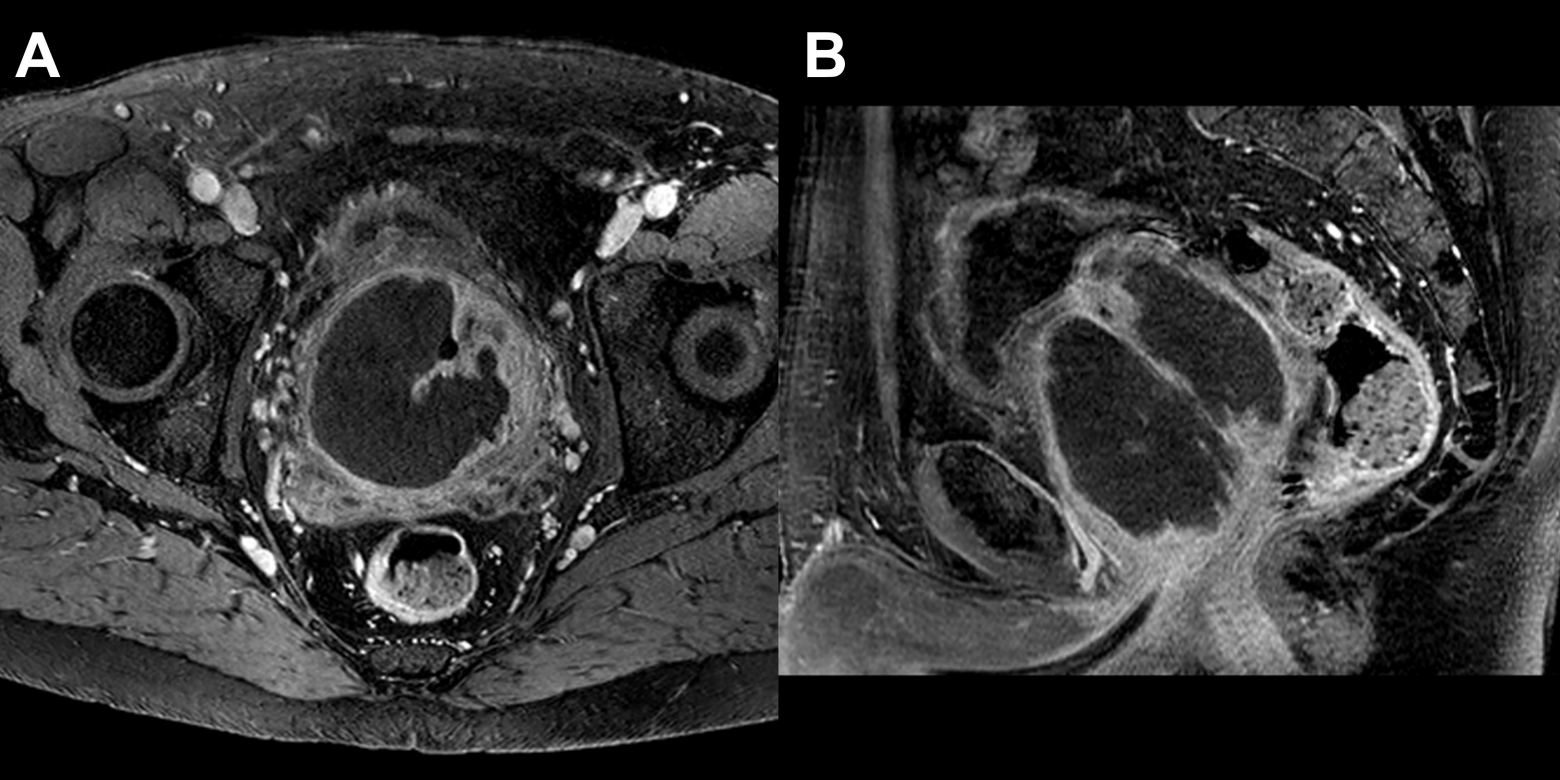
MRI 4-days after PAE: T1 FS after injection of contrast medium shows subtotal devascularization of the transitional zone of the prostate on axial (A) and sagittal (B) planes.
